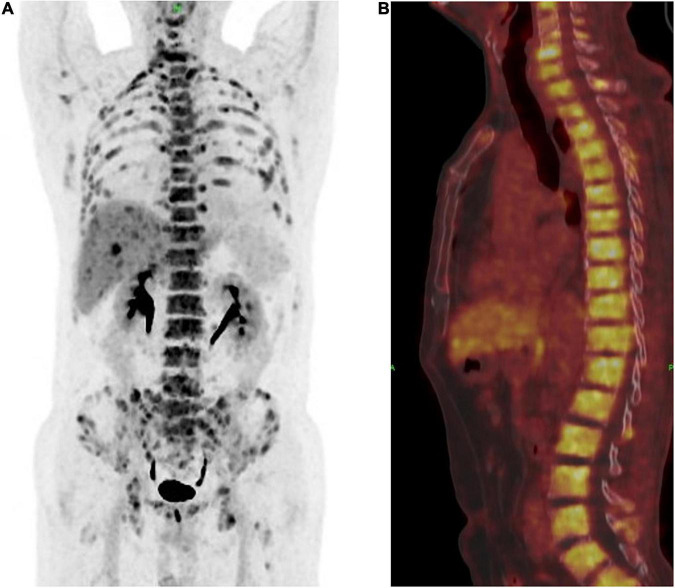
(A) Positron emission tomography and computed tomography (PET-CT) showed a multiple bone destruction with bone metastases including whole vertebra, occipital bone, ribs of double side, appendicular skeleton, and pelvis. (B) PET-CT showed a whole vertebra metastatic Primary EMPD, showing that a most obvious SUV of 18.9.
